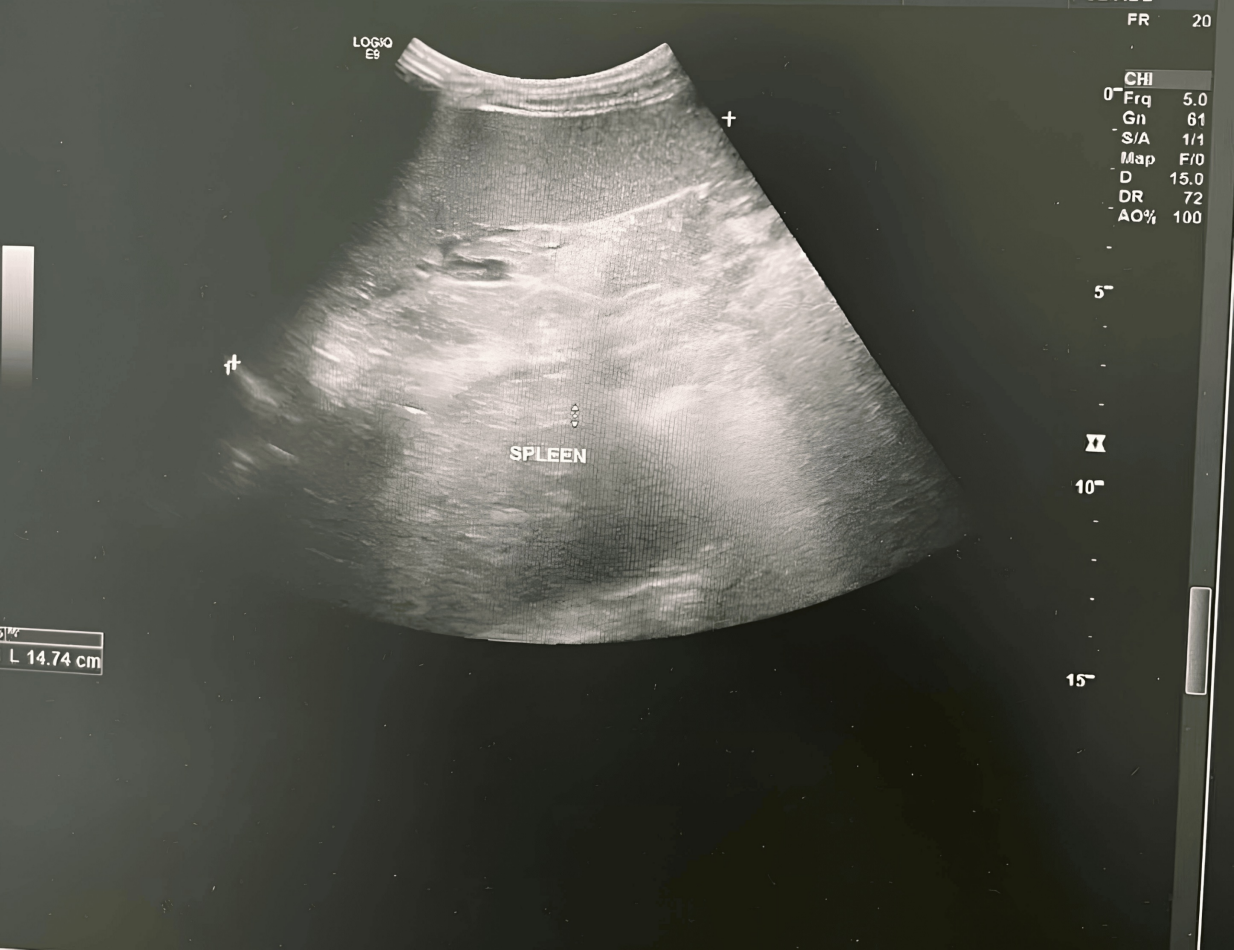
Ultrasound showing spleen size 147mm
